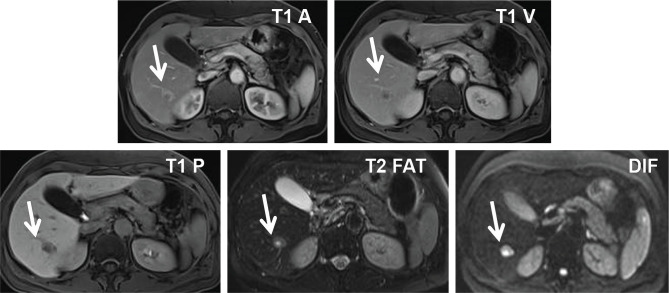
MRI scan, nodule in segment VI showing low intensity on T1-weighted images, high intensity on T2-weighted images, and high intensity in diffusion-weighted images. Images from left to right: T1 arterial, T1 venous, T1 portal, Fat saturated T2, Diffusion.
